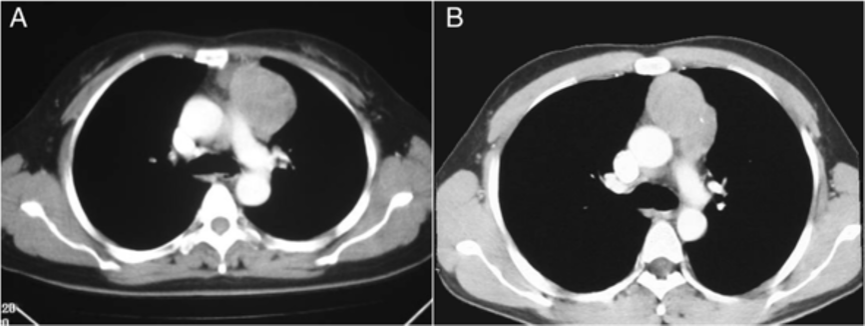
Computed tomography scans of two patients with huge left side thymomas. A: A 43-year-old female patient with a solid tumor located at the left side anterior mediastinum, the diameter of the tumor was 5.6 cm; B: A 56-year-old male patient with a solid tumor located at the left side anterior mediastinum, the diameter of the tumor was 4.2 cm.
